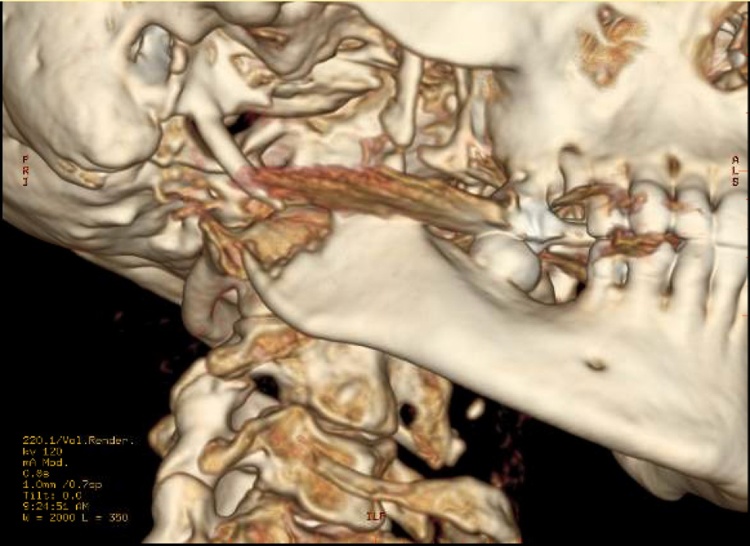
CT scan demonstrating a large enhancing soft tissue mass with central necrosis, measuring 57 × 53 mm.
